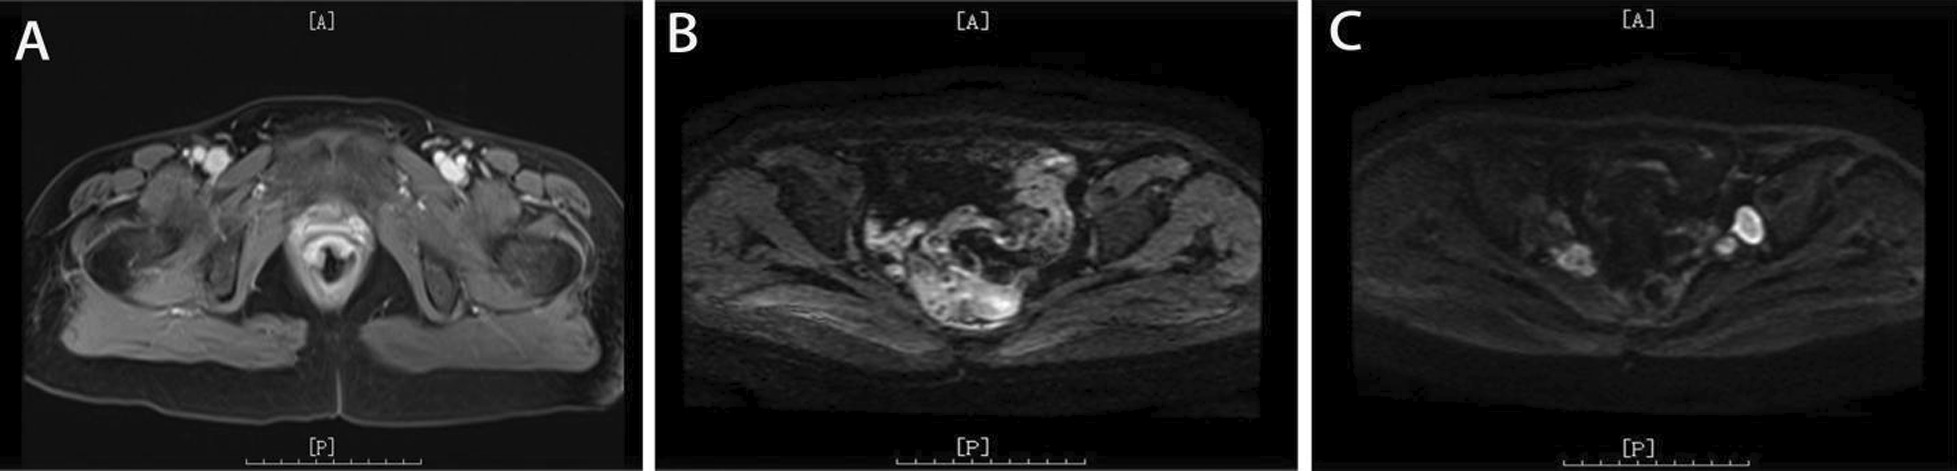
A MRI showed that rectal lesions were enhanced before radical rectal resection. B No recurrence or metastasis was found on MRI during follow-up. C MRI showed that the lateral lymph nodes were enlarged before chemoradiotherapy
